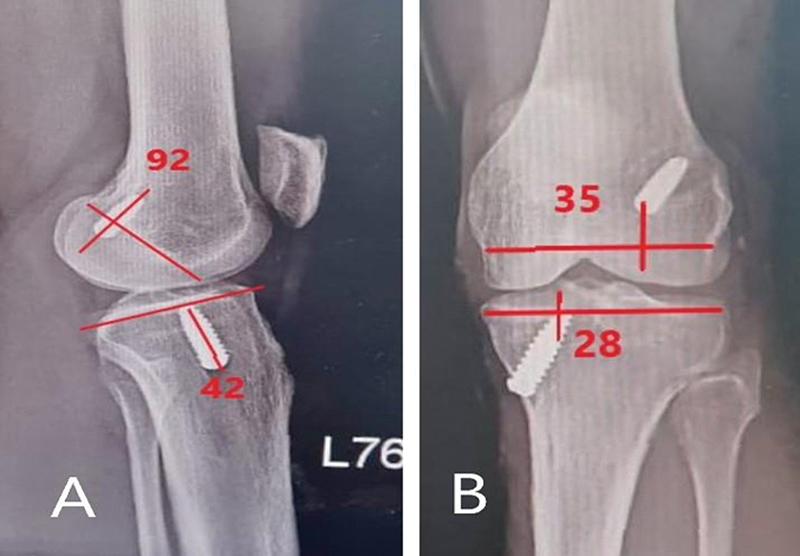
Radiological assessment of tunnel positions in post operative x-ray. A) Coronal femoral and tibial tunnel positions. B) Sagittal femoral and tibial tunnel positions.
